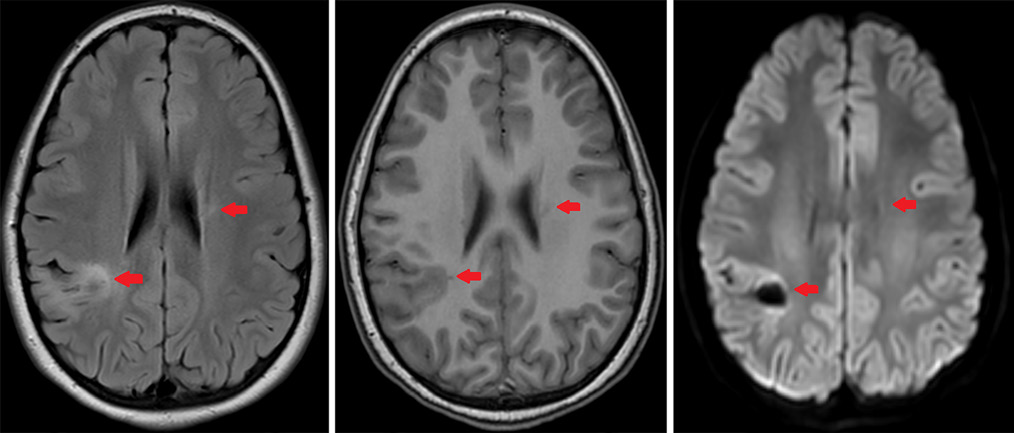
Follow up brain MRI findings. Left: Axial FLAIR image demonstrating decreased FLAIR signal hyperintensity of the white matter lesions (red arrows). Middle: Axial non-contrast T1 image demonstrating lesion signal characteristics relatively isointense to grey matter. Right: Axial DWI image demonstrating decreased diffusion restriction of the lesions. DWI, diffusion-weighted imaging; FLAIR, fluid attenuation inversion recovery.
